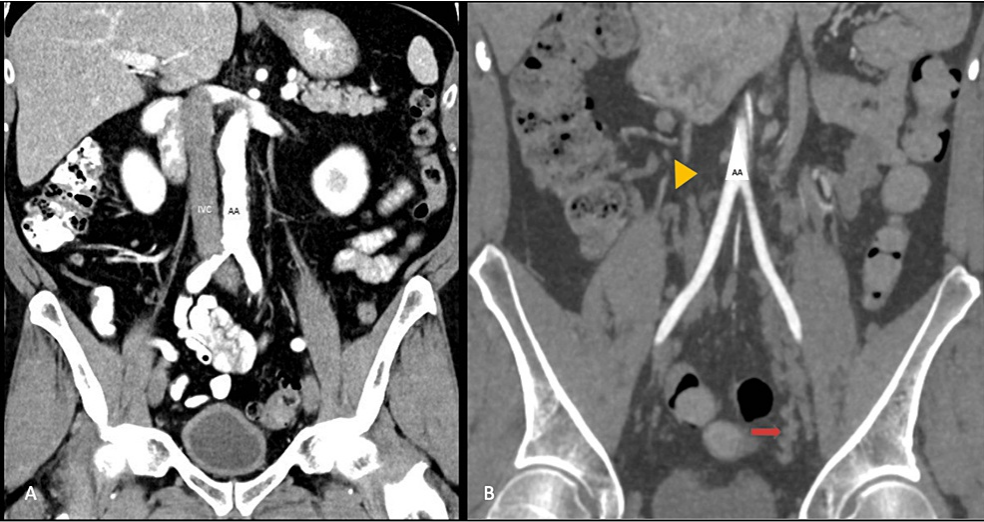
Panel A: CT scan of a normal anatomy with the abdominal aorta (AA) anterior to the inferior vena cava (IVC). Panel B: CT scan with absent IVC (yellow arrowhead) and extensive collateral venous system in the lateral pelvis (red arrow).
